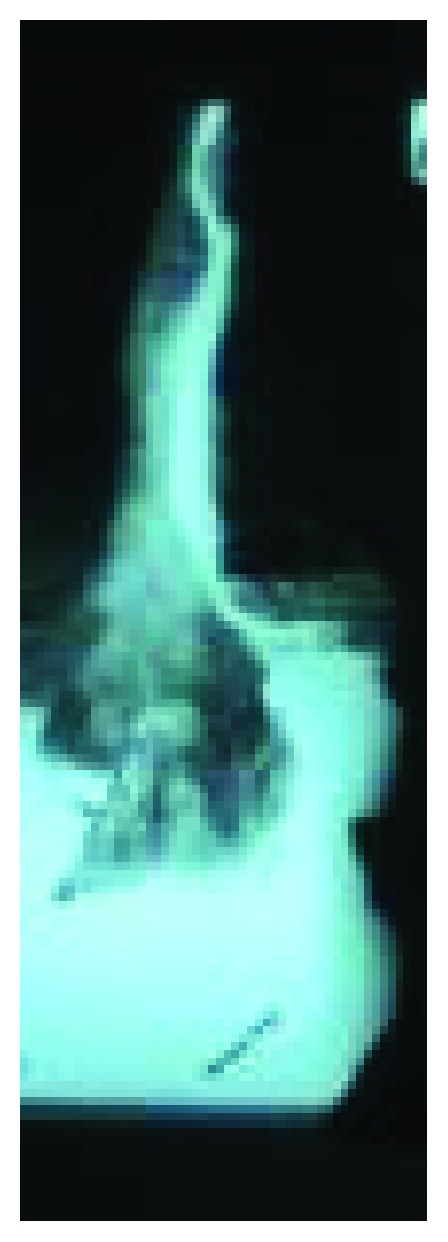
Barium meal X-ray showing stomach extending to the pelvis.
